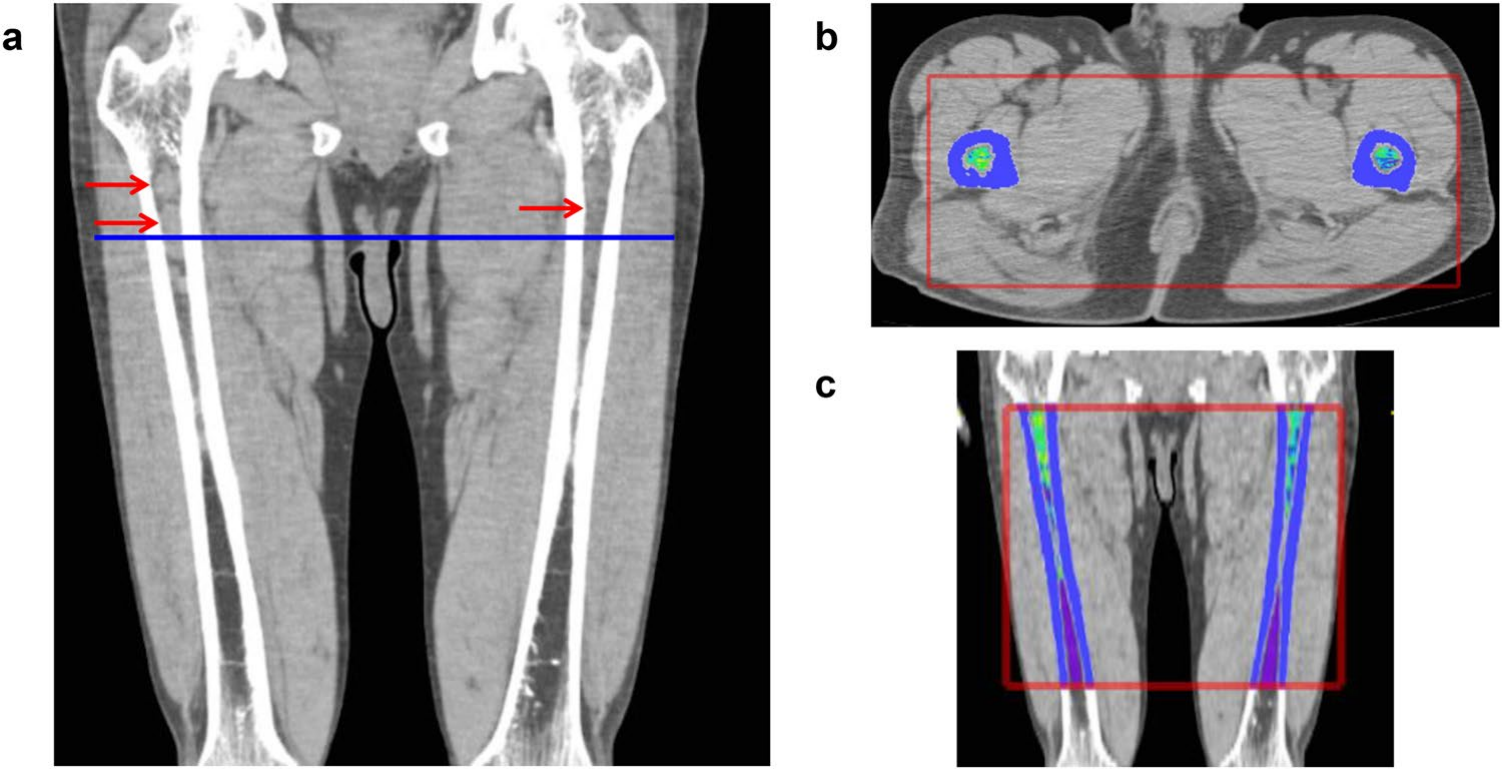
Representative images of a patient with advanced IgAκ myeloma. (a) Coronal CT image of the femora. Myeloma infiltration was detected in the upper metaphysis and diaphysis in both the left and right femora (arrows). (b) Axial image at the level of the blue line shown in (a). The software provides pseudo-colour according to the Hounsfield unit (HU) (high: yellow-red, low: blue-purple). (c) Coronal image reconstructed from the axial slices. Red rectangle: horizontal and vertical ranges for the determination of cumulative CT value (cCTv). The cCTv of this case was 4.46 HU.
